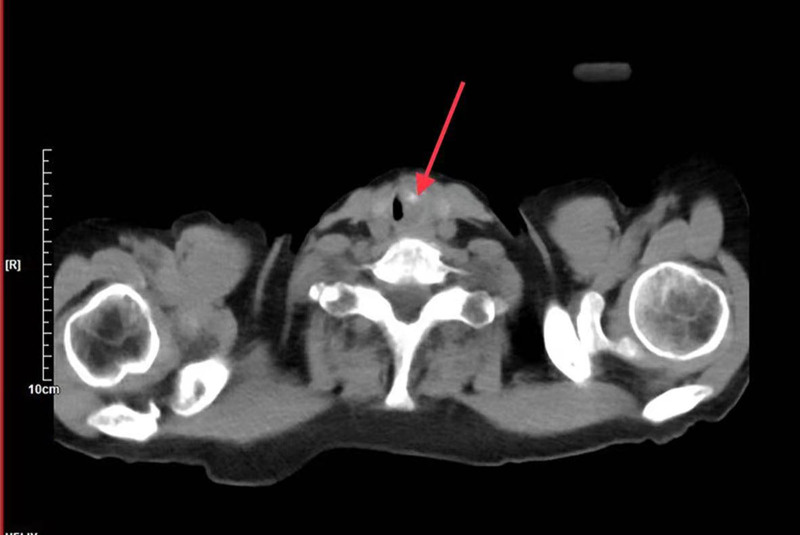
CT examination showed soft tissue shadow with calcification on the left posterior wall of the initial tracheal segment, with local tracheal cavity stenosis accounting for about 80% (red arrow).
